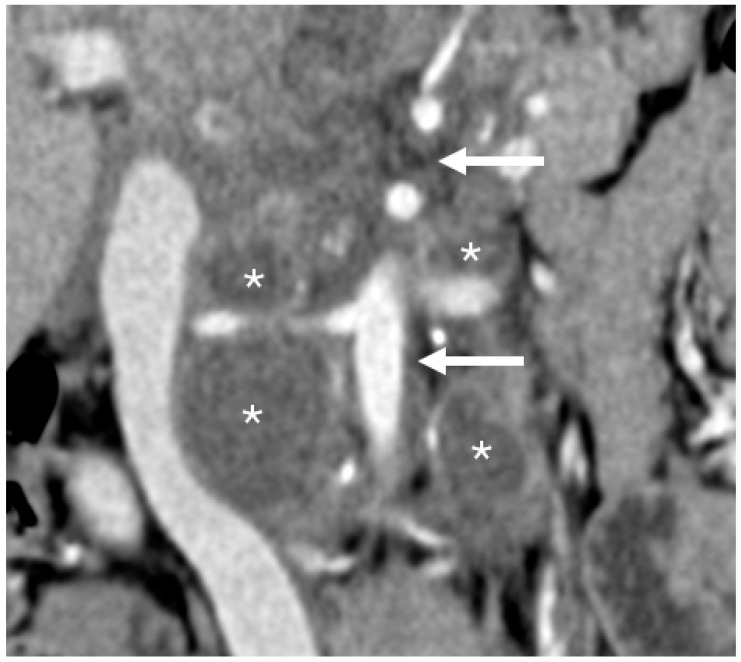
Coronal post-contrast CT image of a 5-year-old girl with neuroblastoma showing multiple retroperitoneal nodules (asterisks). In addition, there are areas of increased attenuation of the fat surrounding the aorta and its branches (arrows), without discrete margins, calcification, or mass effect. While these abnormalities may reflect post-chemotherapy changes rather than tumor, they should still be modeled as they can pose challenges to resection.
